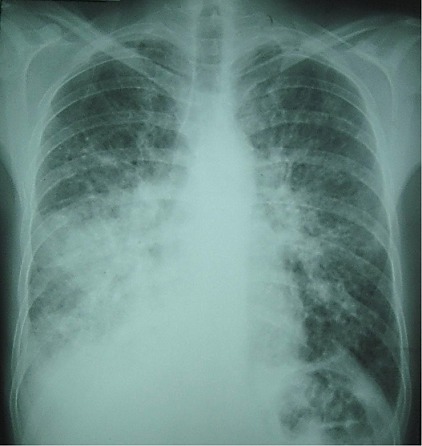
Radiographie thoracique montrant des opacités réticulo-nodulaires bilatérales, confluentes par endroit et prédominantes à la base droite et à la région hilaire gauche
